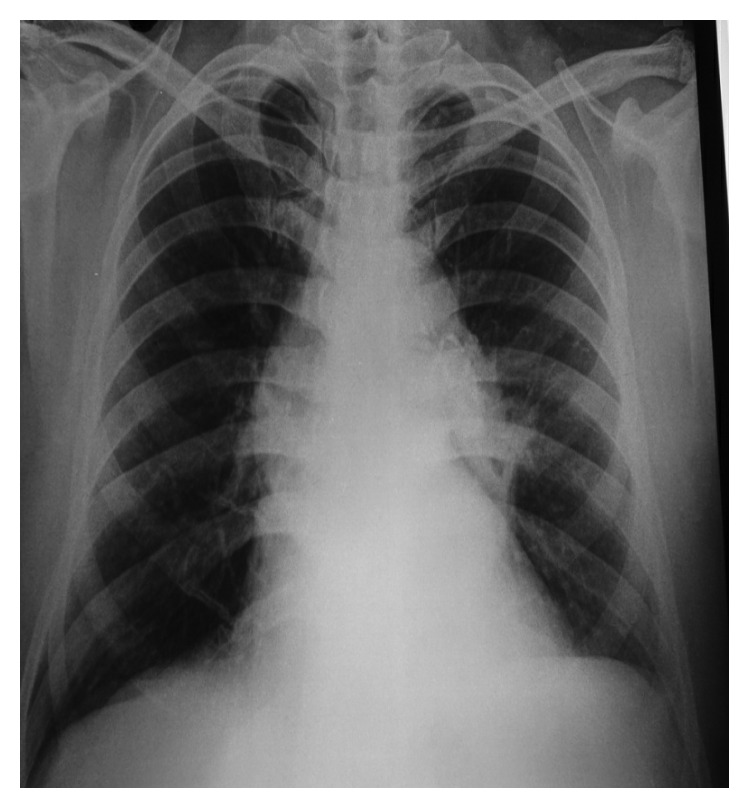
Chest X-ray revealed raised left hemidiaphragm and left midzone opacity, creating a positive silhouette sign with the left cardiac border.
